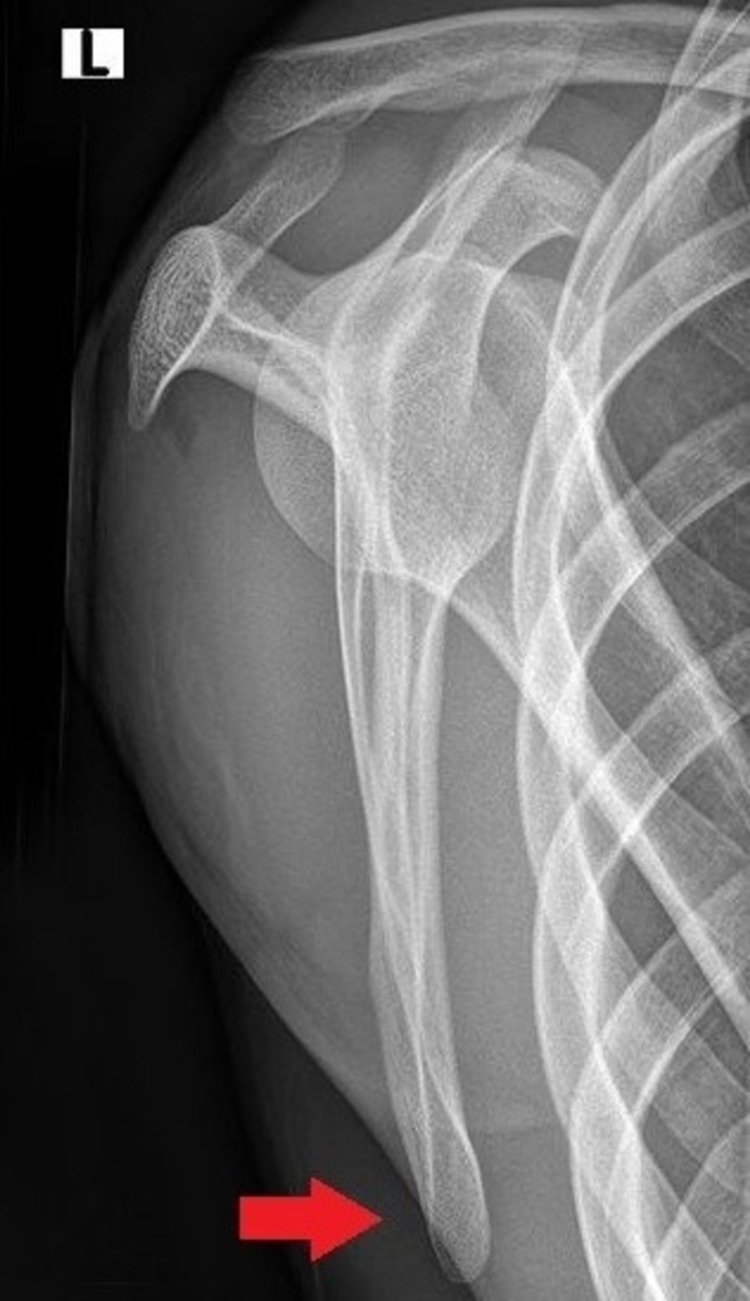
Lateral x-ray at three-month follow-up revealed complete fracture healing (red arrow)
